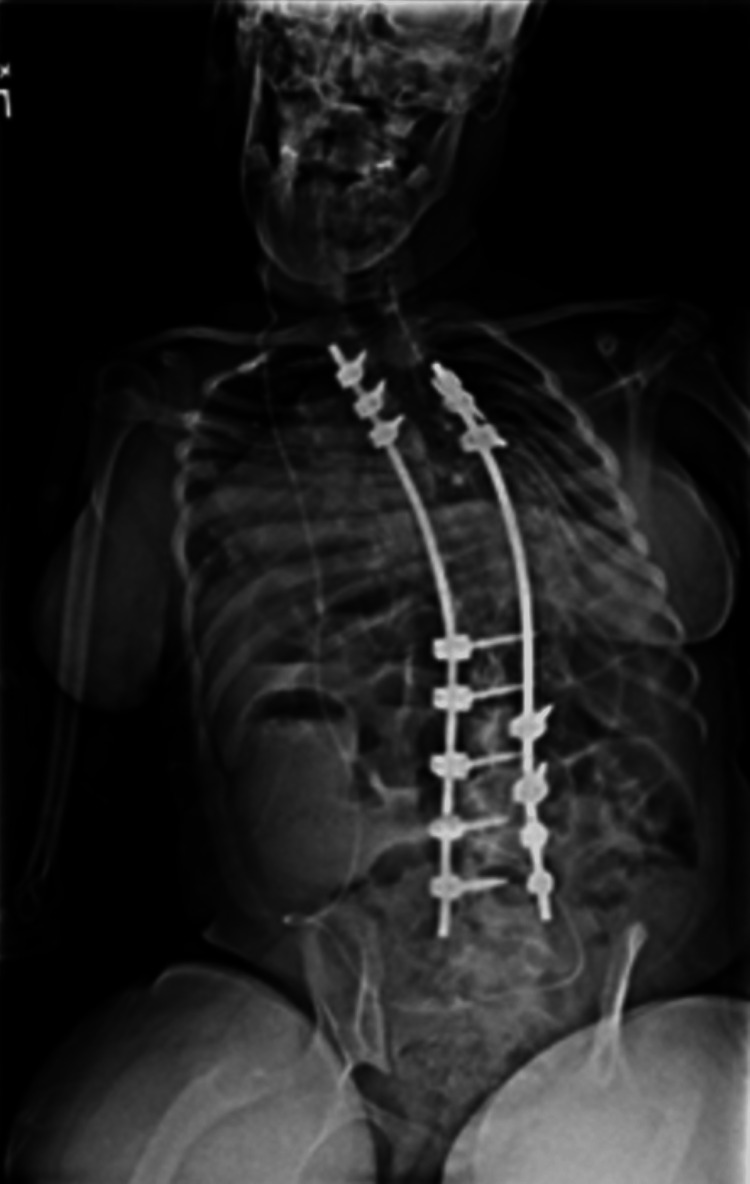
AP plain film performed after conversion surgery from MAGEC rods to fixed cobalt chrome rods.
